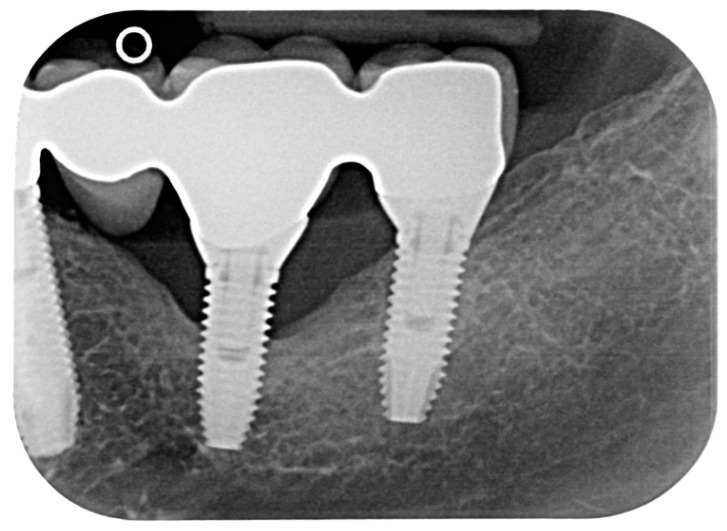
Periapical radiograph of one case of peri-implantitis in a non-diabetic subject.
